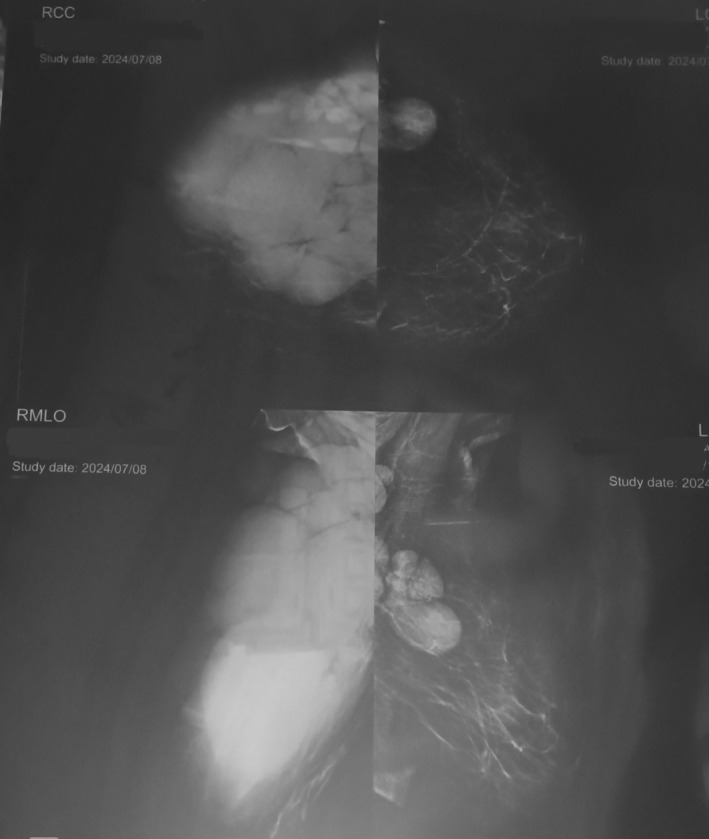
MRI evaluation of breast involvement by CLL showed a giant lobulated hyperdense mass in the right breast, which occupies almost all of the breast and axillary space. Also, a similar, smaller lobulated mass in the upper outer quadrant of the left breast was seen.
